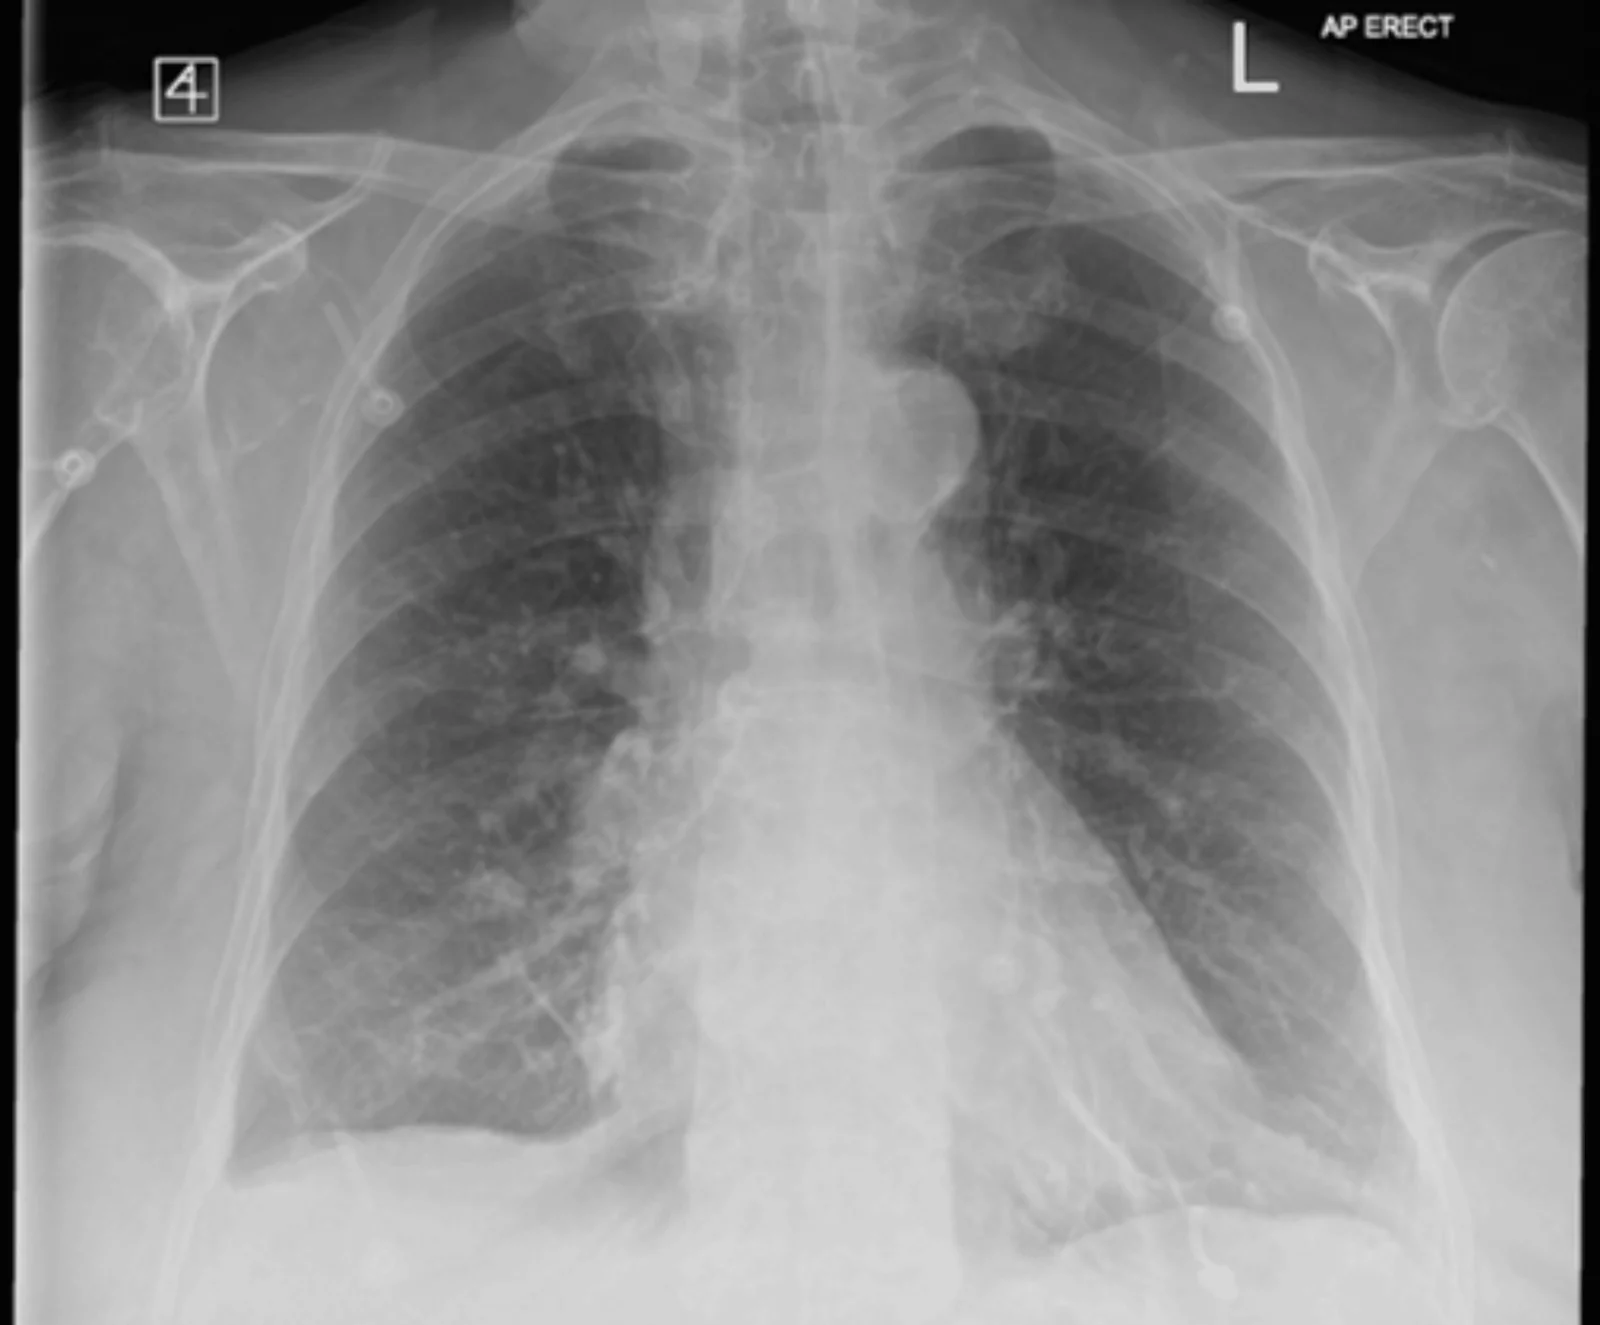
Chest X-ray No pneumoperitoneum identified.
